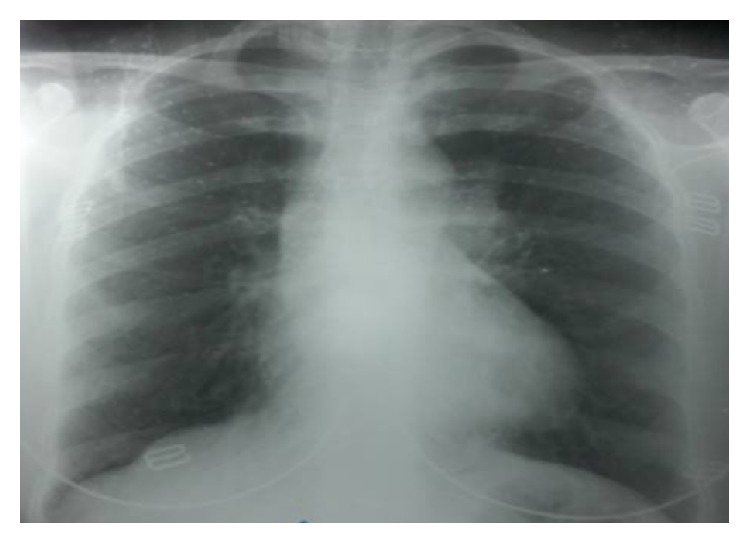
Posteroanterior chest X-ray showing a mild increase in cardiothoracic ratio.
